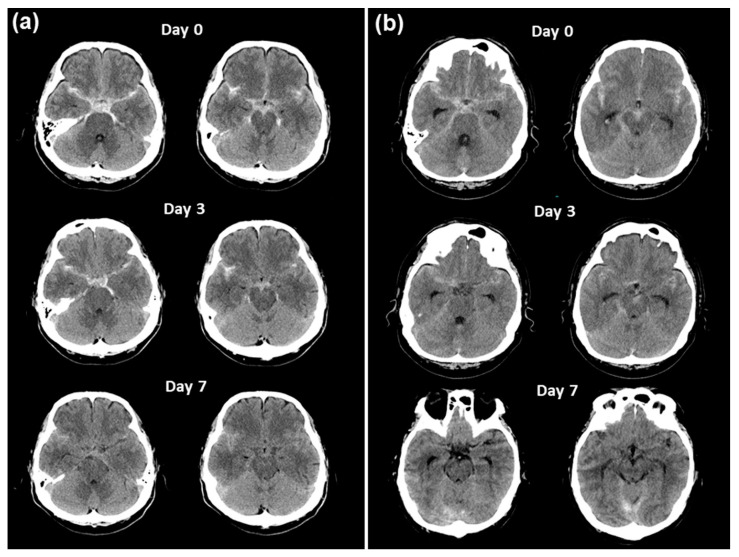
Serial brain CT imaging in the present case (a) with severe vasospasm on day 9 and a contracting case (b) with good clinical course without vasospasm following ruptured VADA.
